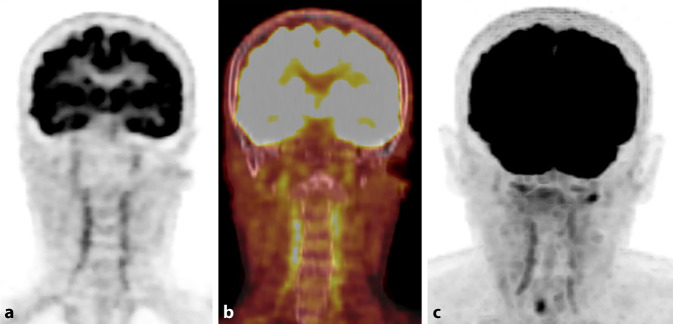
a [18F]-FDG-PET, koronar, „standardized uptake value“ (SUV) 7; b [18F]-FDG-PET/CT, koronar, Fusionsbild, SUV 5; c [18F]-FDG-PET, koronar, Maximumintensitätsprojektion (MIP), SUV 7. Deutliche Stoffwechselsteigerung der A. vertebralis beidseits
